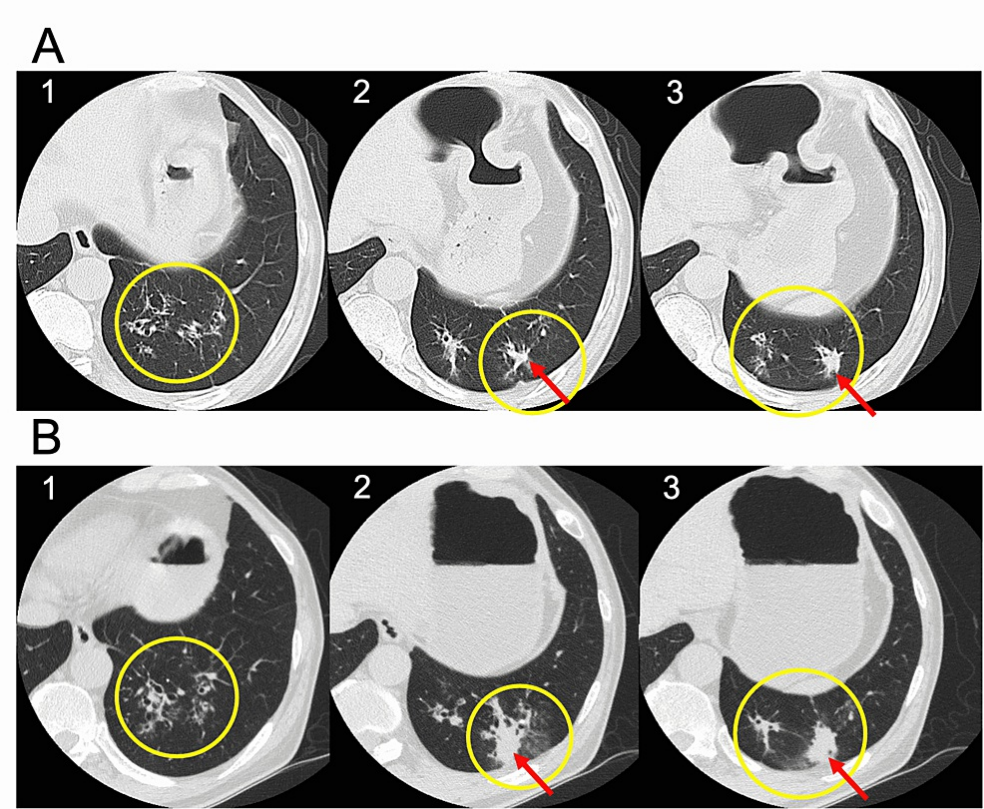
Chest computed tomography on the first examination (A) and on the admission for a bronchoscopy (B) In four months from the first examination (A) to the admission for a bronchoscopy (B), infiltrative and ground glass shadows around bronchiectasis (1) and nodules (2, 3) worsened (yellow circles), and nodular lesions increased in size (2, 3) (red arrows) in the left lower lung lobe.
